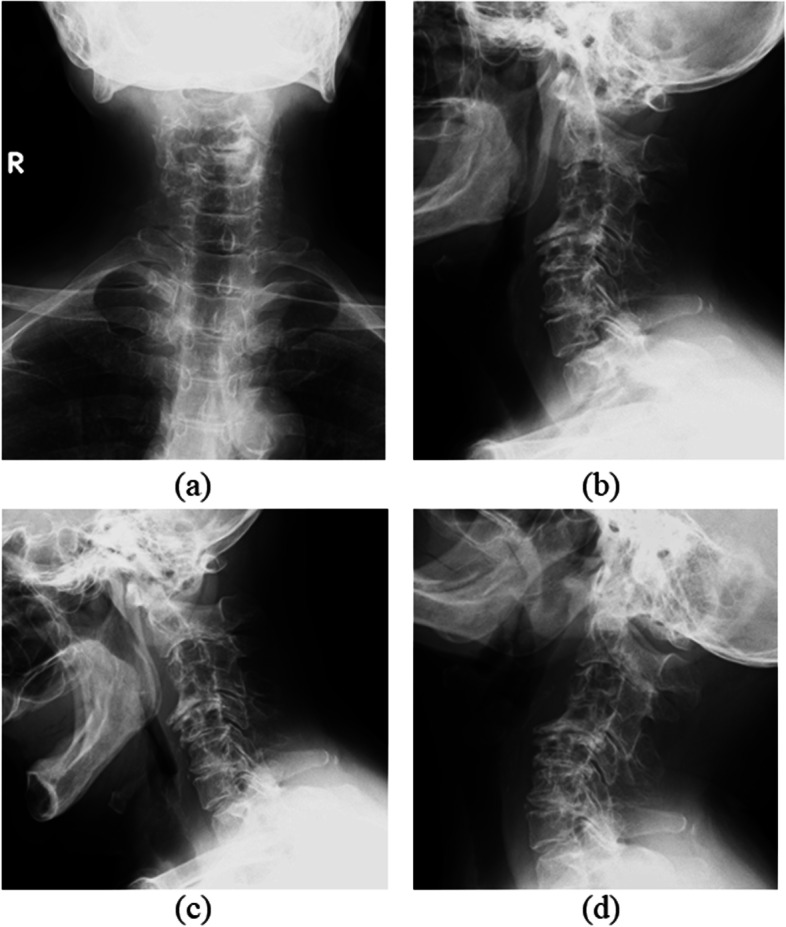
Preoperative radiographs. Radiographs showed osteolytic lesions in C3–7
